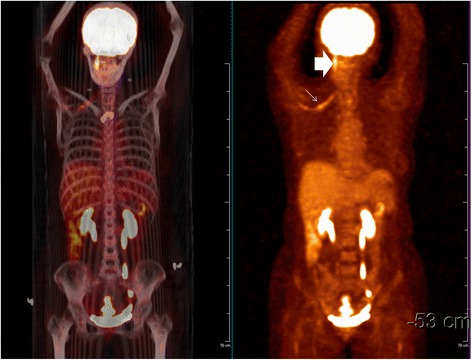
PET/CT before treatment showing involvement of right vagus nerve and brachial plexus (whole body images). Wide white arrow indicates high glucose metabolism area of the right vagus nerve running zone; fine white arrow indicates high glucose metabolism area of the right brachial plexus running zone
